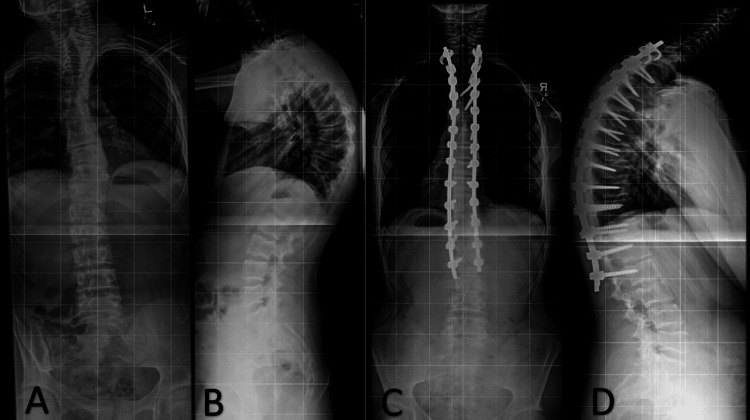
Case four preoperative and postoperative imaging. 36-inch, standing X-ray obtained preoperatively (A - standing posteroanterior X-ray; B - lateral X-ray) and postoperatively (C - standing PA X-ray; D - lateral X-ray). Improved thoracic kyphosis and scoliosis can be seen in images A & B to C & D.
